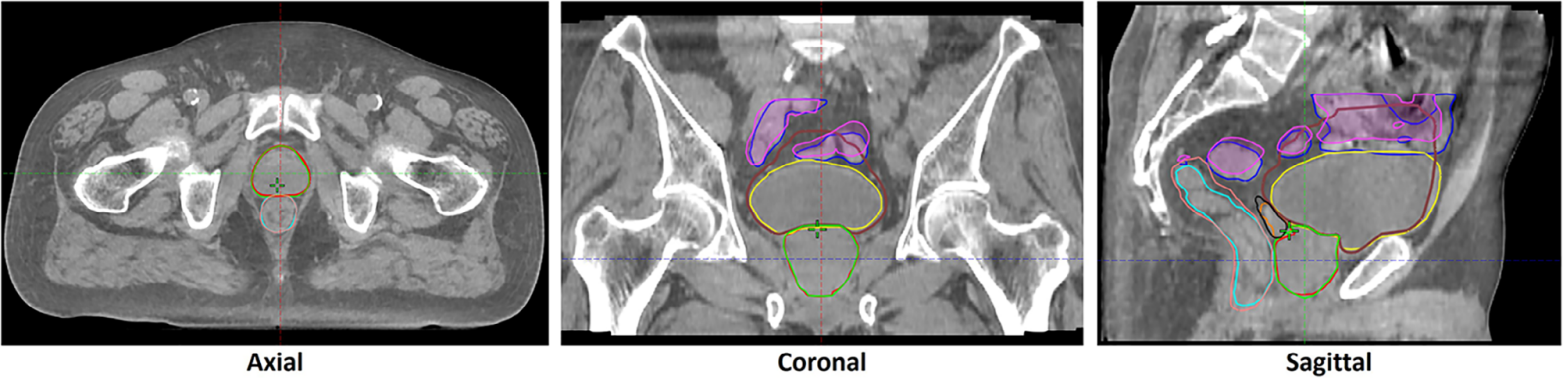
Intra‐fractional variation in contours between two CBCT scans, CBCT1 vs CBCT2. Prostate (red vs green), seminal vesicles (orange vs black), bladder (yellow vs brown), rectum (cyan vs pink), and bowel (blue vs purple). CBCT, cone beam computed tomography.
